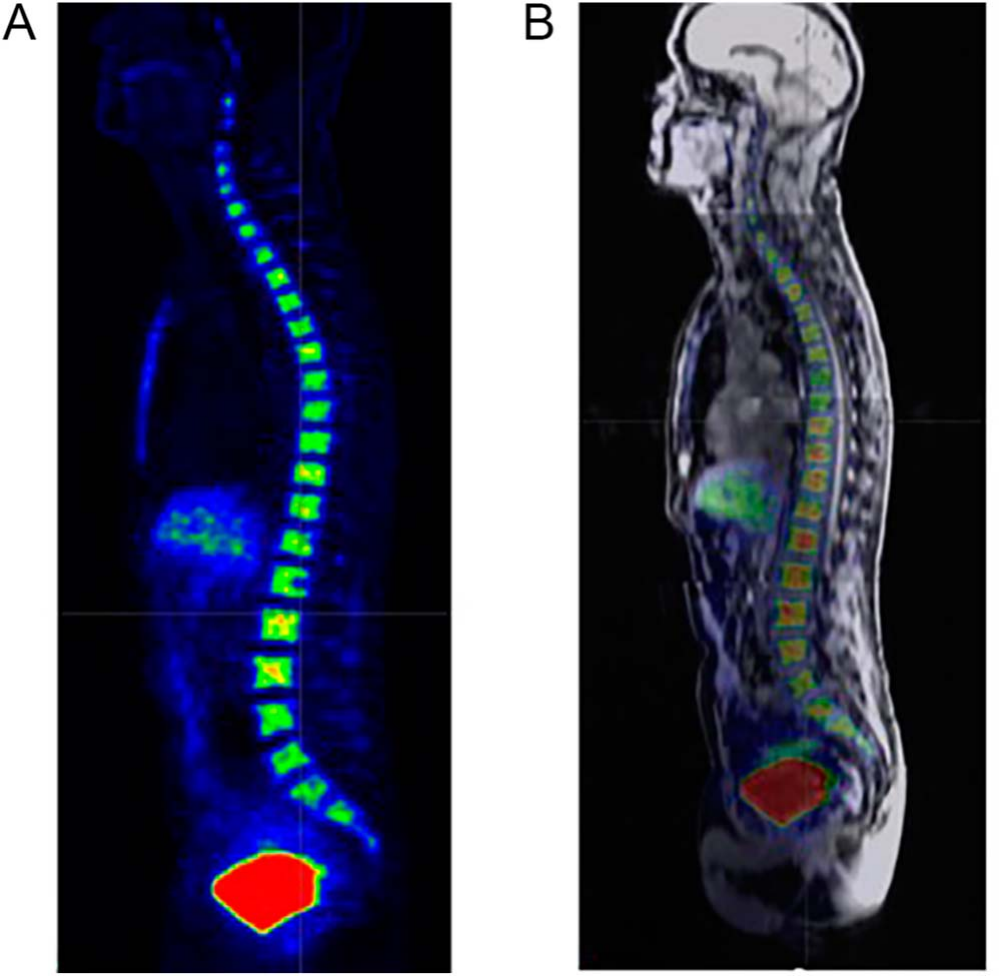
Sagittal image of pure ^18^F-FLT PET information (A) and fused sagittal ^18^F-FLT PET/MR image (B).
